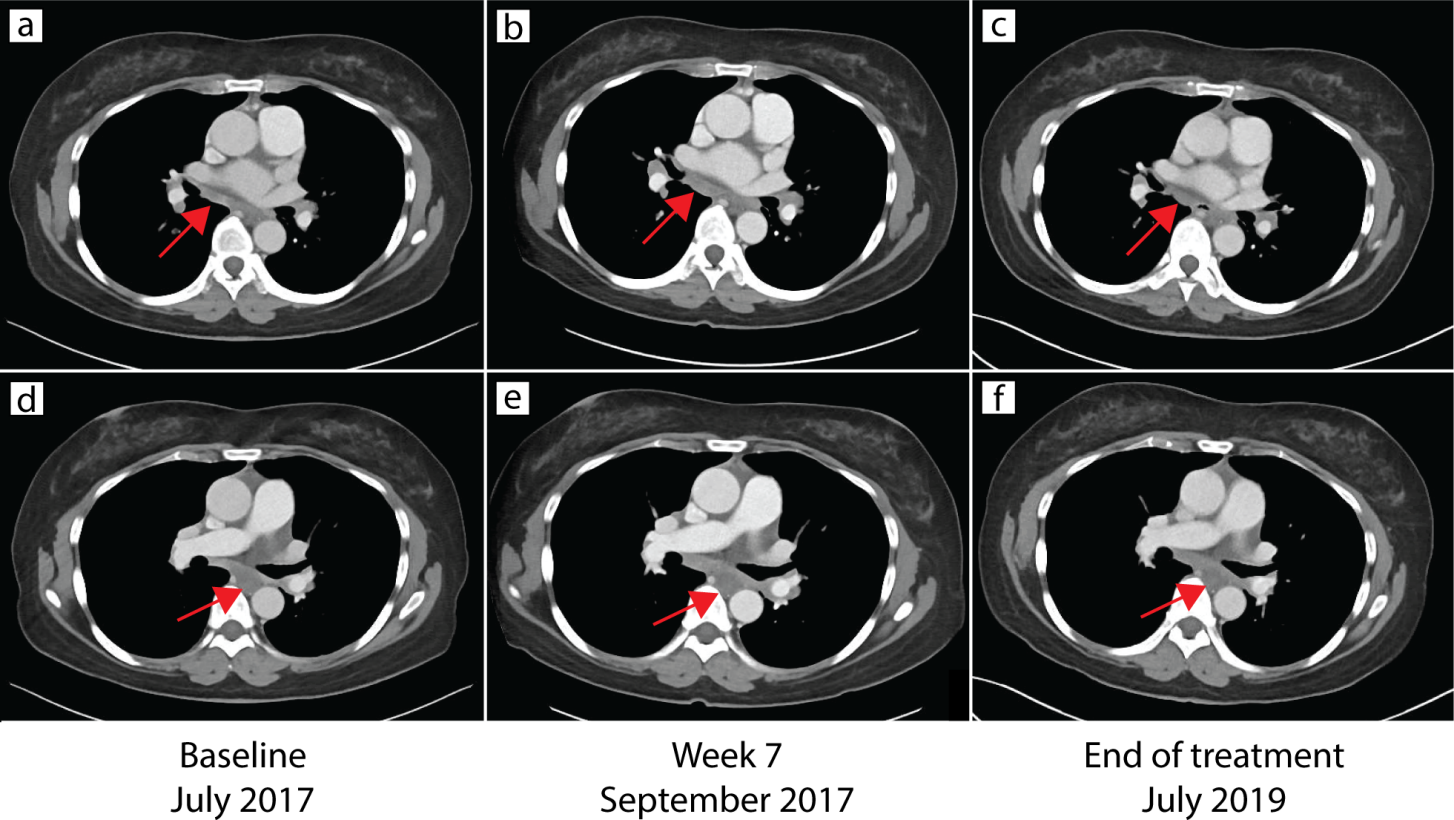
CT of chest demonstrating stable mediastinal lymphadenopathy. CT of the chest at baseline in July 2017 (a, d), week 7 in September 2017 (b, e), and end of treatment in July 2019 (c, f) demonstrated stable mediastinal lymphadenopathy (arrows).
